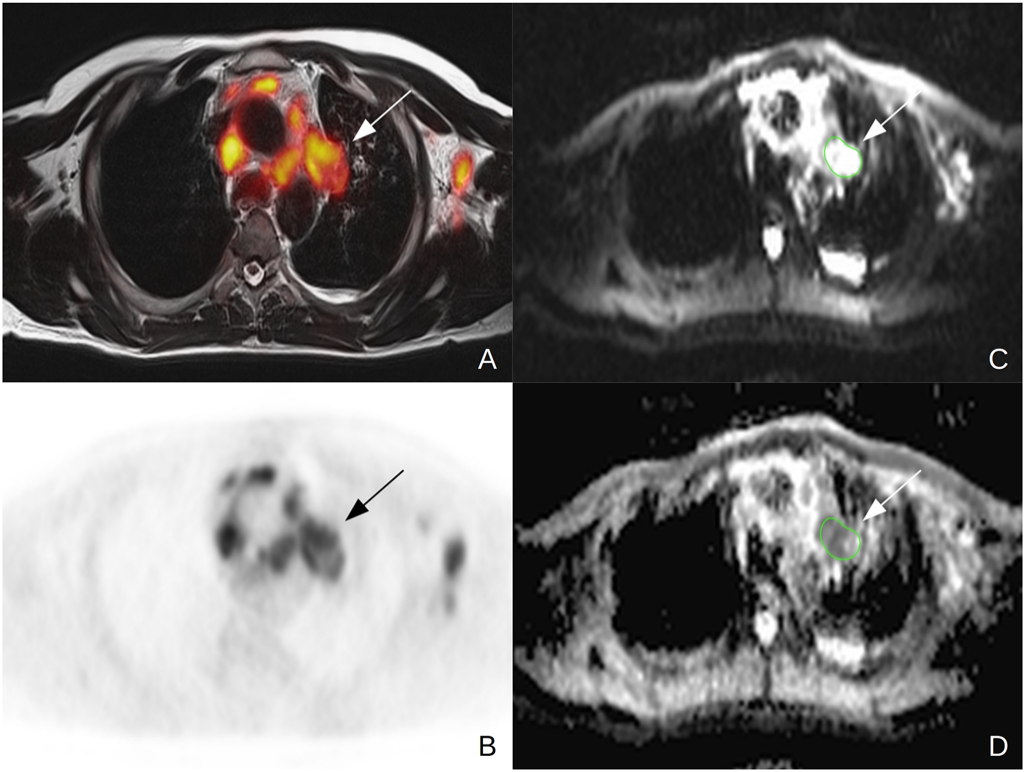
44y old male patient suffering from NSCLC (tumor stage IV, adenocarcinoma, unknown tumor grading) Note the FDG-avid lymph node in the aortopulmonary window in the fused PET/MR (A) and PET (B) images. For ADC_mean_ evaluation, a polyginal ROI was drawn around the lymph node on the b0 image in DWI and then copied to the ADCmap.
